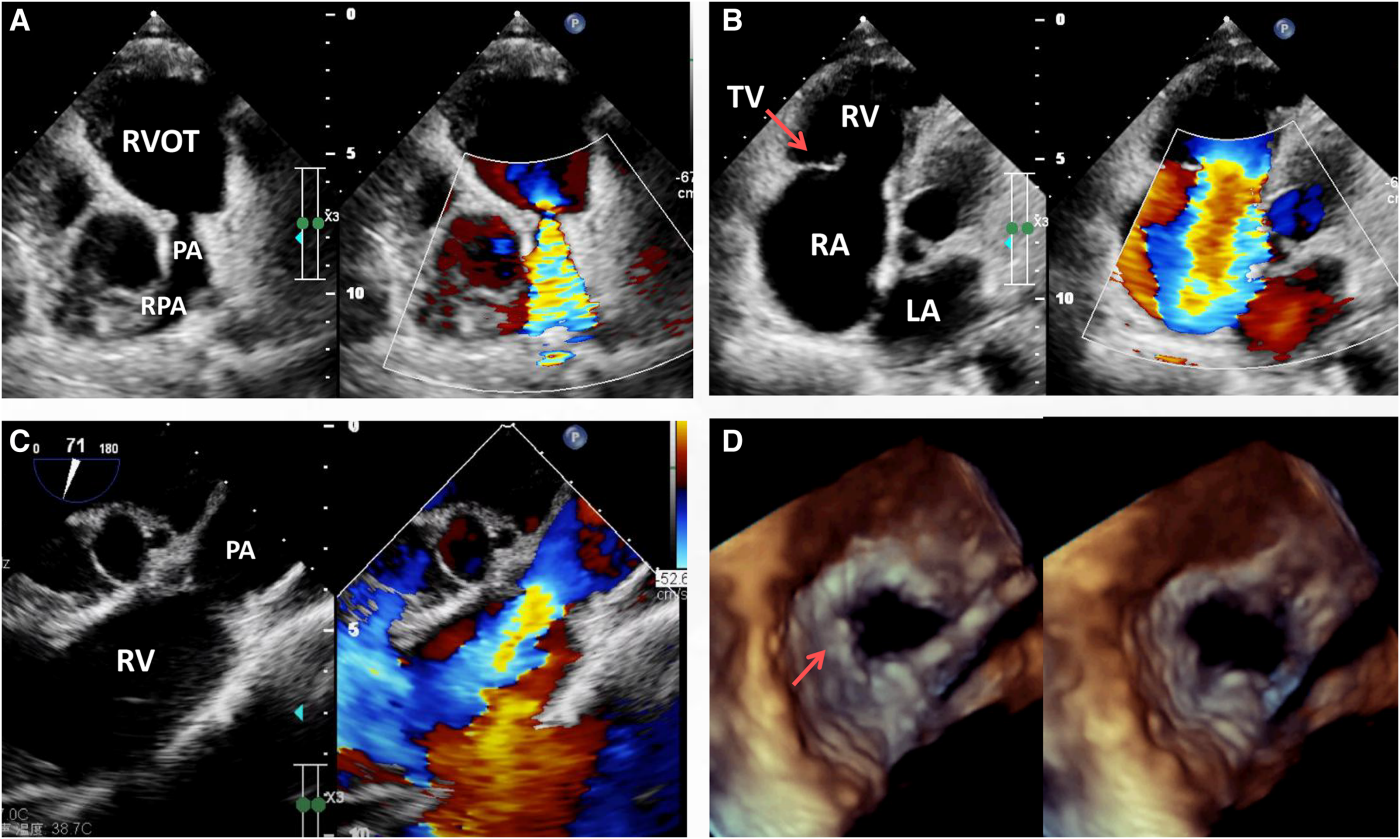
Transthoracic echocardiogram (TTE) pre-operation revealing the presence of severe pulmonary stenosis with the contracture of the pulmonary annulus and valves but with no sign of RVOT stenosis (A), RA enlargement, severe tricuspid regurgitation (B, arrow) and pulmonary regurgitation (C). Transesophageal echocardiography (TEE) immediately before surgery revealing severe pulmonary valvular insufficiency in the cardiac end diastolic period and end systolic period (D, arrow). LA, left atrium; PA, pulmonary artery; RA, right atrium; RPA, right pulmonary artery; RV, right ventricle; RVOT, right ventricular outflow tract; TV, tricuspid valve.
